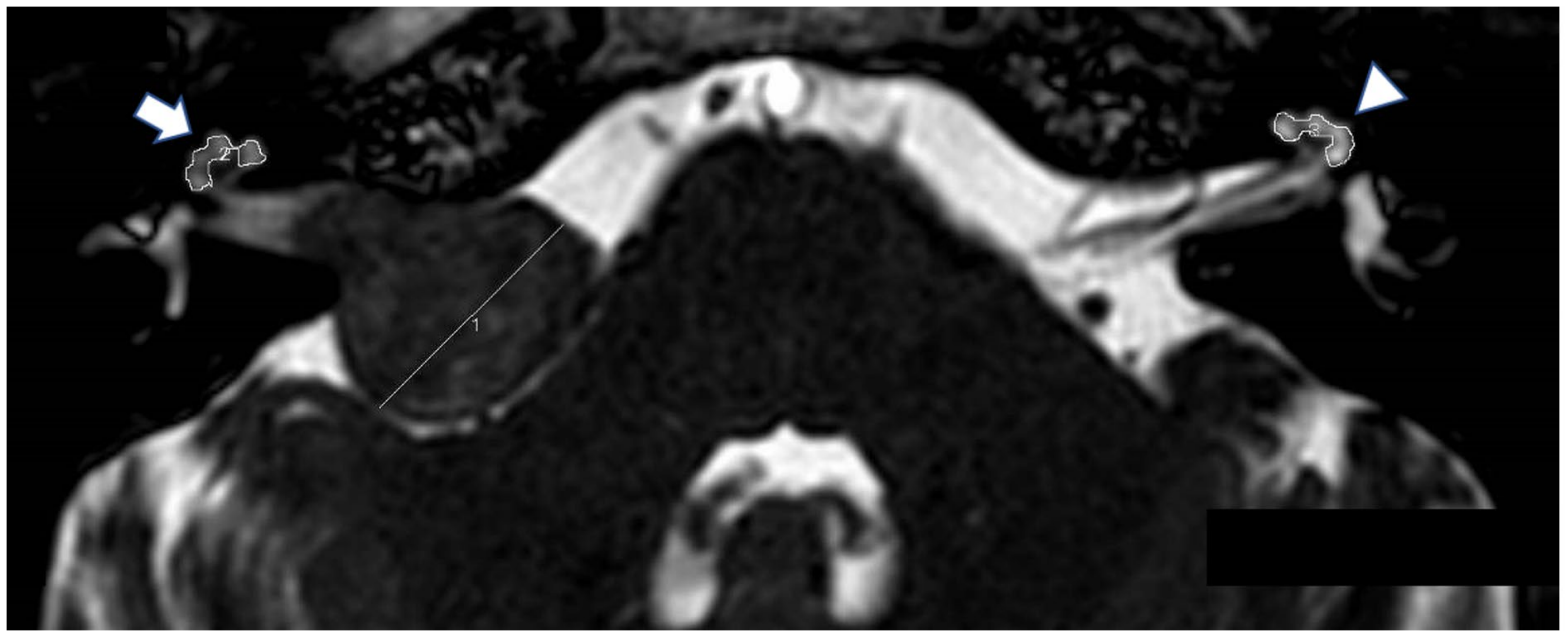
Balanced fast field-echo (bFFE) sequence showing regions of interest (ROIs) and the tumor diameter. The MRI image displays hand-drawn ROIs around the affected cochlea (arrow) and the normal cochlea (arrowhead).
